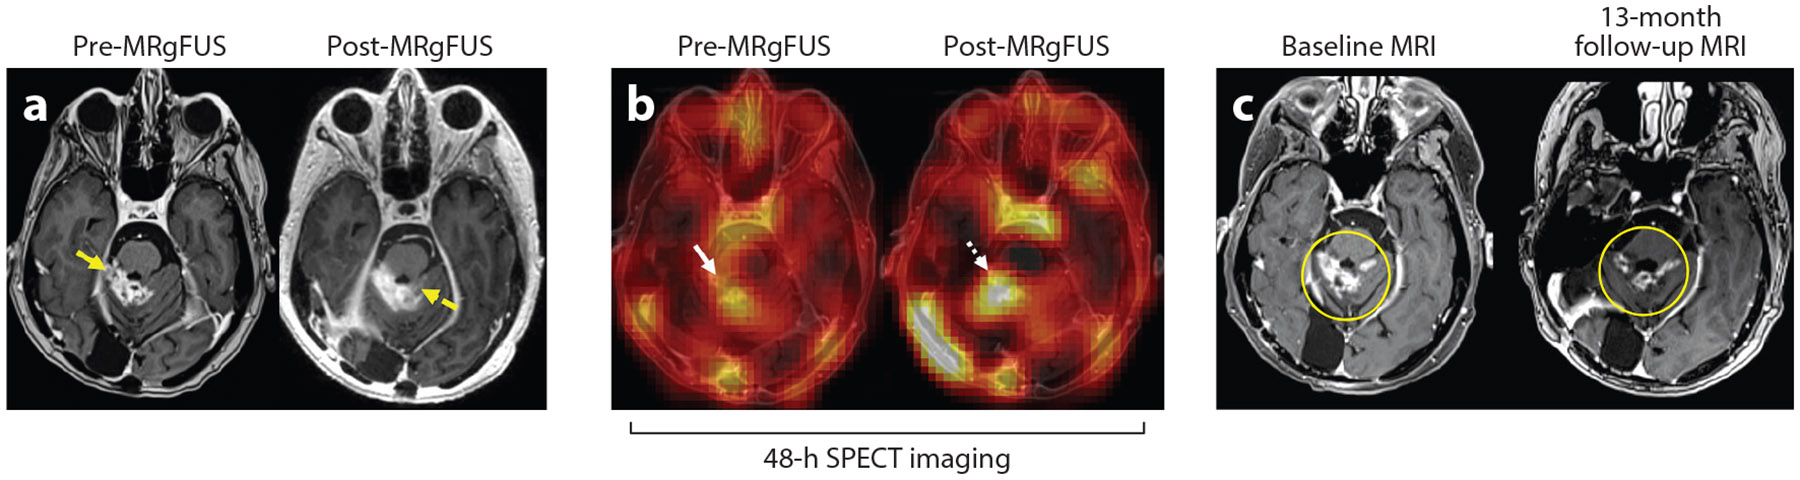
Visual demonstration of antibody delivery across the BBB/BTB of BCBM with MRgFUS. (*a*) Depictions of BCBM on T1-weighted MRI pre- and post-MRgFUS BBB/BTB-O. Yellow arrows denote contrast enhancement in the tumor and surrounding region before and after sonication. (*b*) SPECT imaging 48 h pre- and post-MRgFUS BBB/BTB-O following delivery of ^111^In-BzDTPA-NLS-trastuzumab. White arrows denote increase in the SPECT signal in the region targeted with FUS, suggesting increased antibody delivery. (*c*) BCBM (*yellow circle*) on baseline scan prior to therapeutic intervention and on follow-up scan taken 13 months after treatment. Follow-up imaging exhibits reduction in tumor burden 13 months after FUS + trastuzumab treatment. Abbreviations: BBB, blood–brain barrier; BBB/BTB-O, BBB/BTB opening; BCBM, breast cancer brain metastasis; BTB, blood–tumor barrier; FUS, focused ultrasound; MRgFUS, magnetic resonance–guided FUS; MRI, magnetic resonance imaging; SPECT, single-photon emission computed tomography. Figure adapted with permission from Reference 62.
